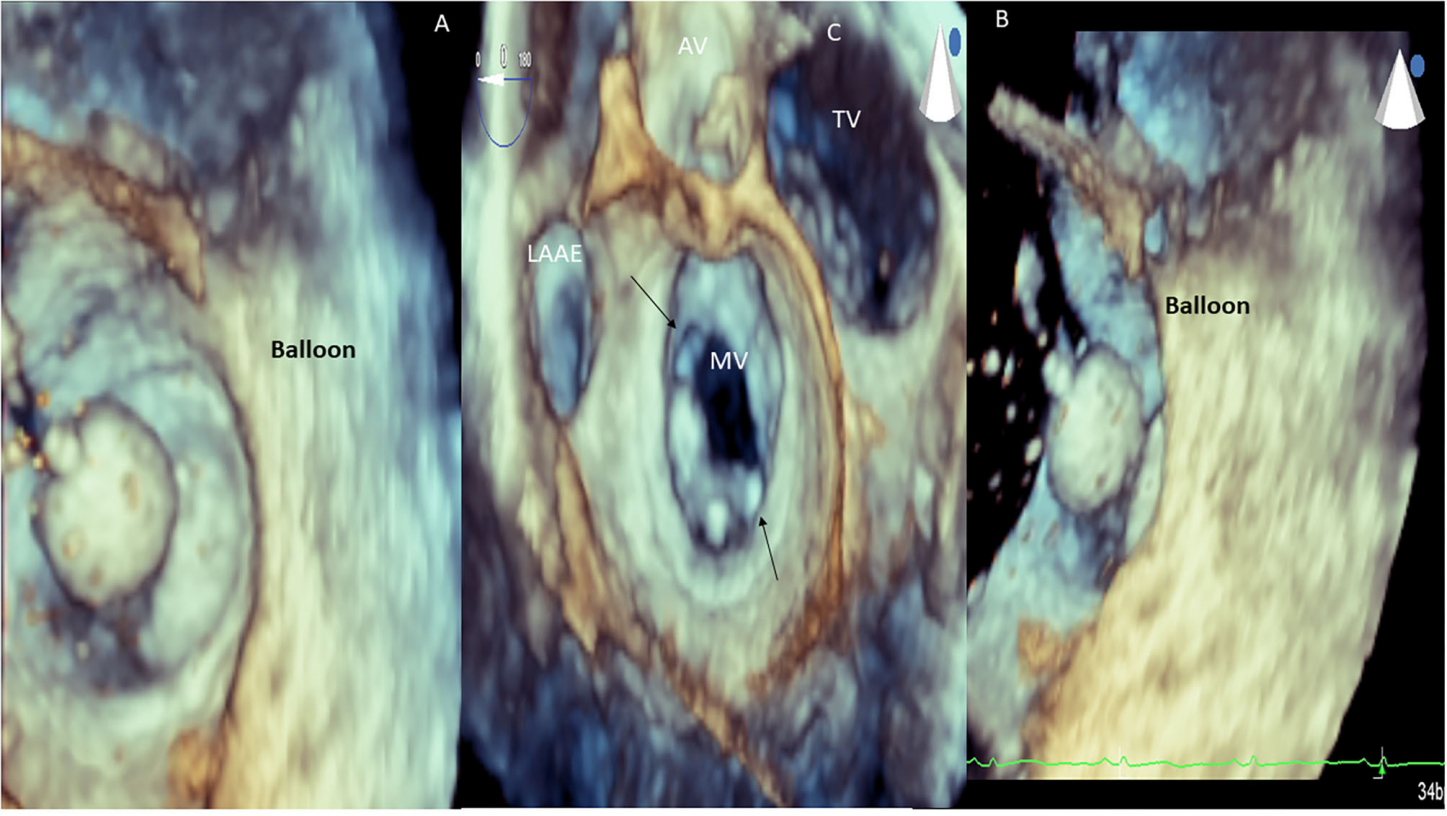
RHD (mitral valve stenosis). 3D transesophageal echocardiography. Demonstration of balloon valvuloplasty in patient with severe mitral valve stenosis (A,B). 3D transesophageal echocardiography. Demonstration of mitral valve commissural opening (arrows, C) after balloon valvuloplasty. LAAE, left atrium appendage; AV, aortic valve; MV, mitral valve; TR, tricuspid valve.
